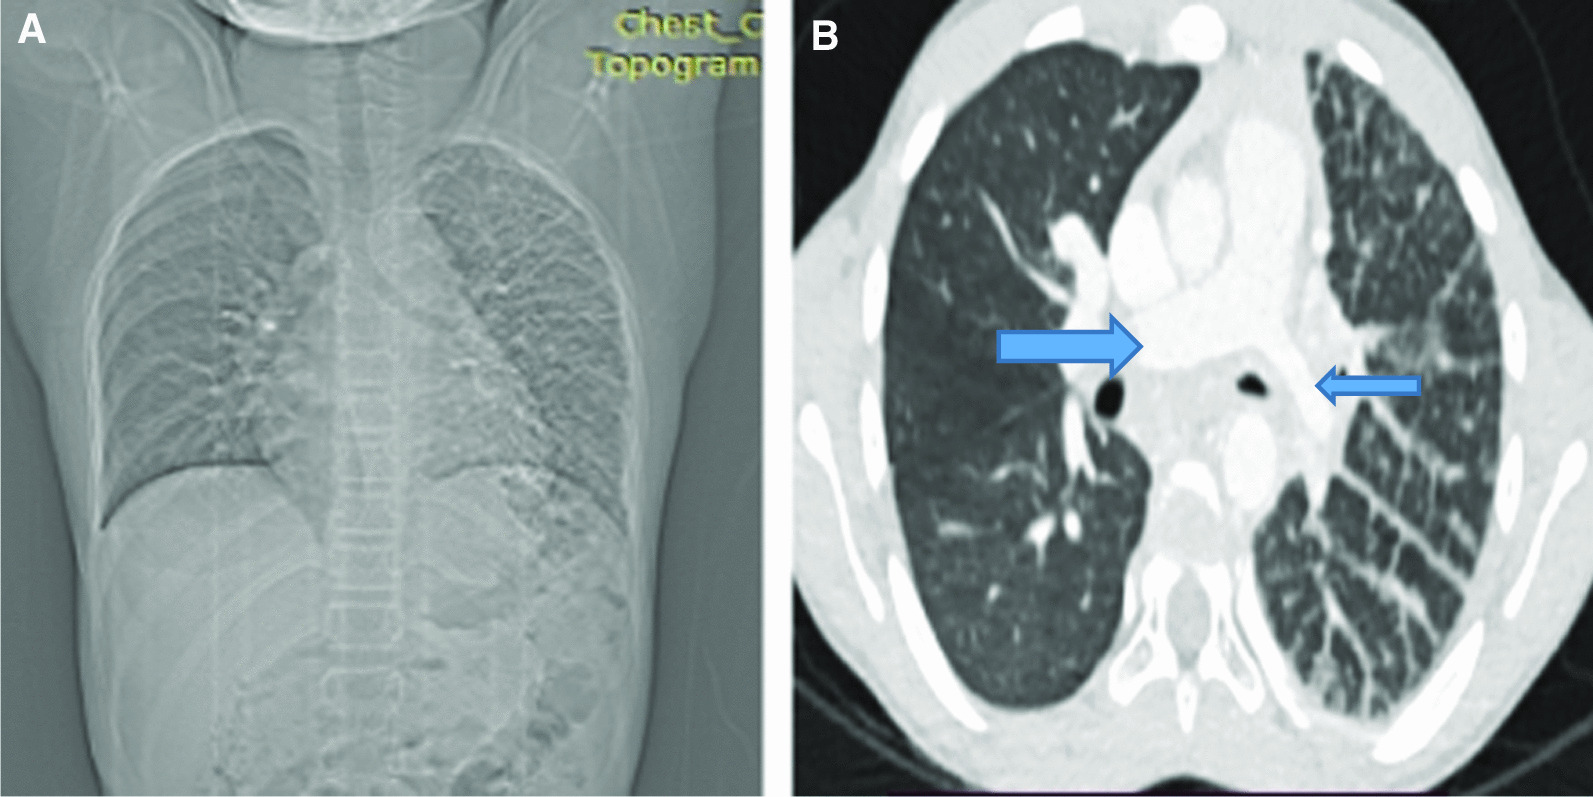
A Postero Anterior chest x-ray (PA CXR). Left hemithorax appears smaller and diffuse reticulation with smaller hilum. Right hemithorax is normal. B Contrast enhanced chest Computed tomography showing left lung diffuse interlobular septal thickening and ground glass opacity with a normal right lung. Note the significantly smaller left pulmonary artery (smaller arrow) as compared to the right (bigger arrow)
